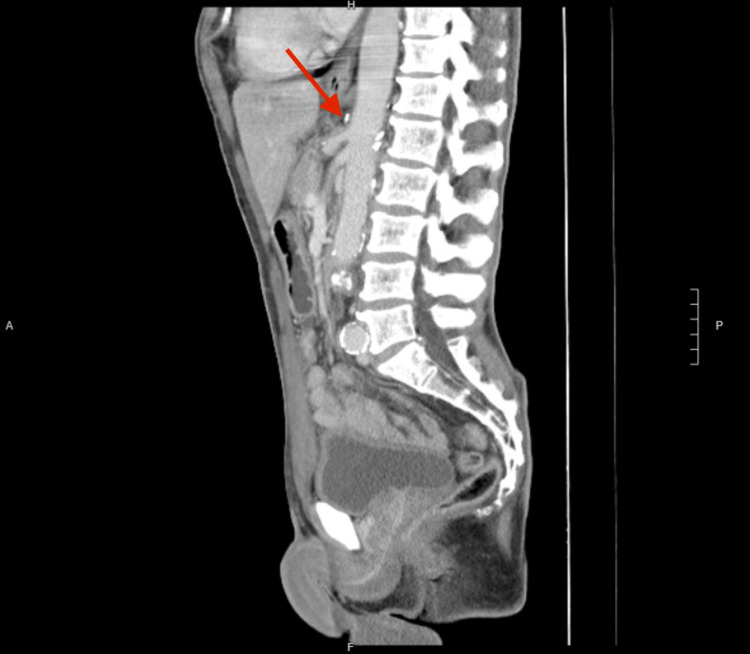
Postoperative CT scan of the abdomen/pelvis showing no evidence of celiac artery compression. CT: computed tomography
